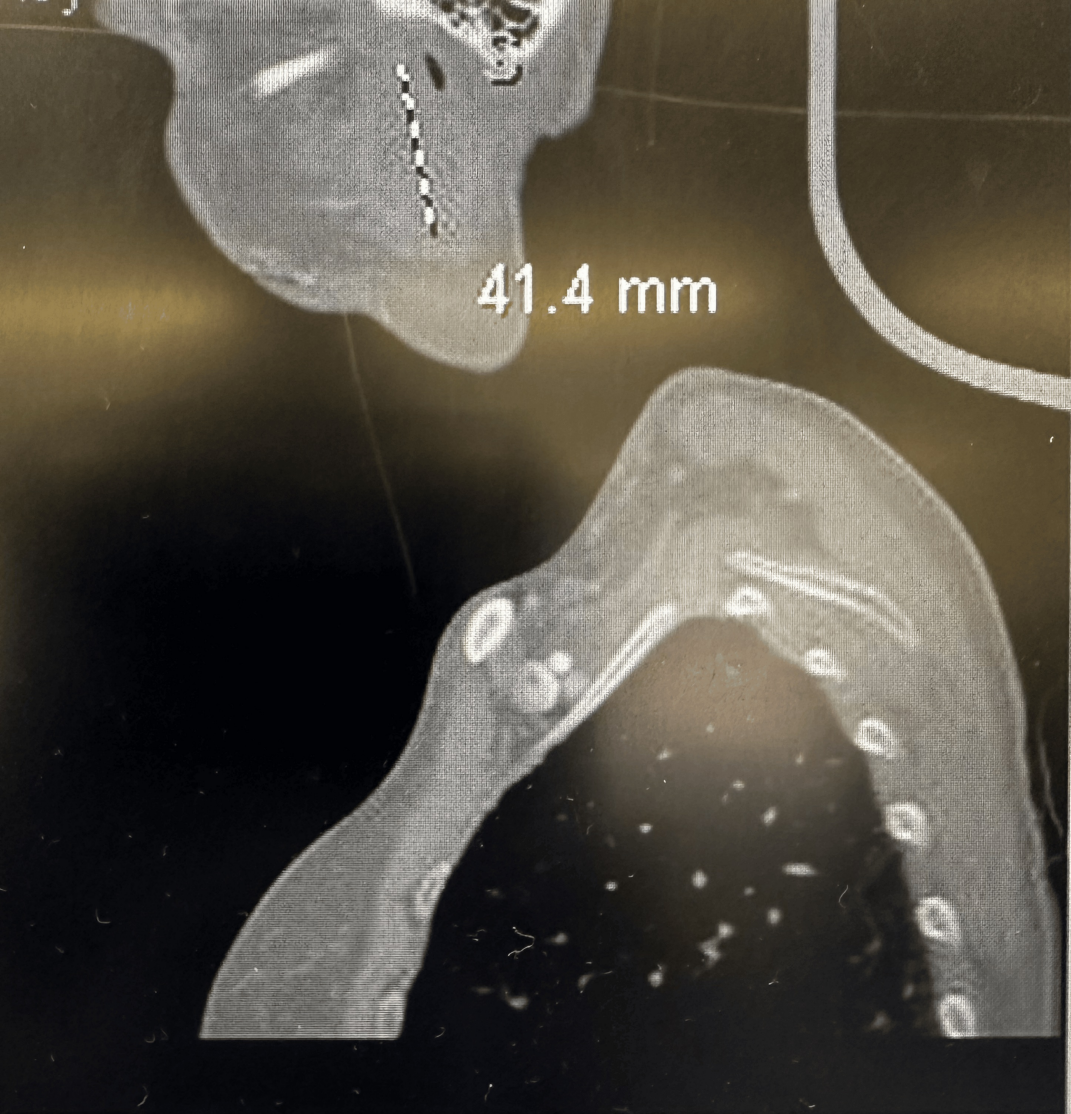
CT soft tissue neck CT soft tissue neck (sagittal view) showed right superficial parotid nodule measuring up to 4.1 cm
